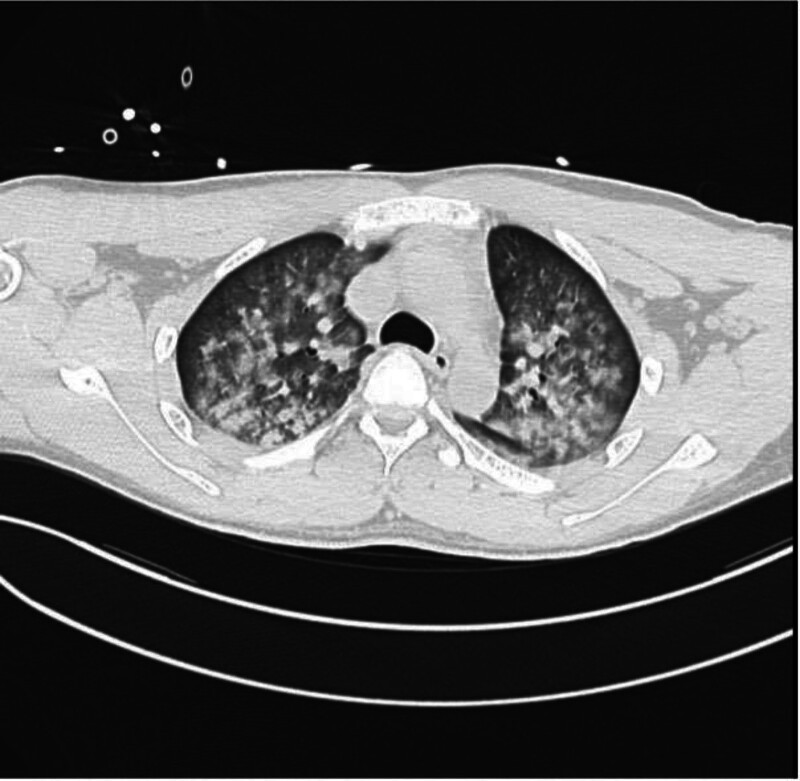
Non-contrast chest CT (May 15, 2025): extensive bilateral ground-glass opacities with peri-hilar and upper lobe predominance, 6 hours post-extubation. CT = computed tomography.
